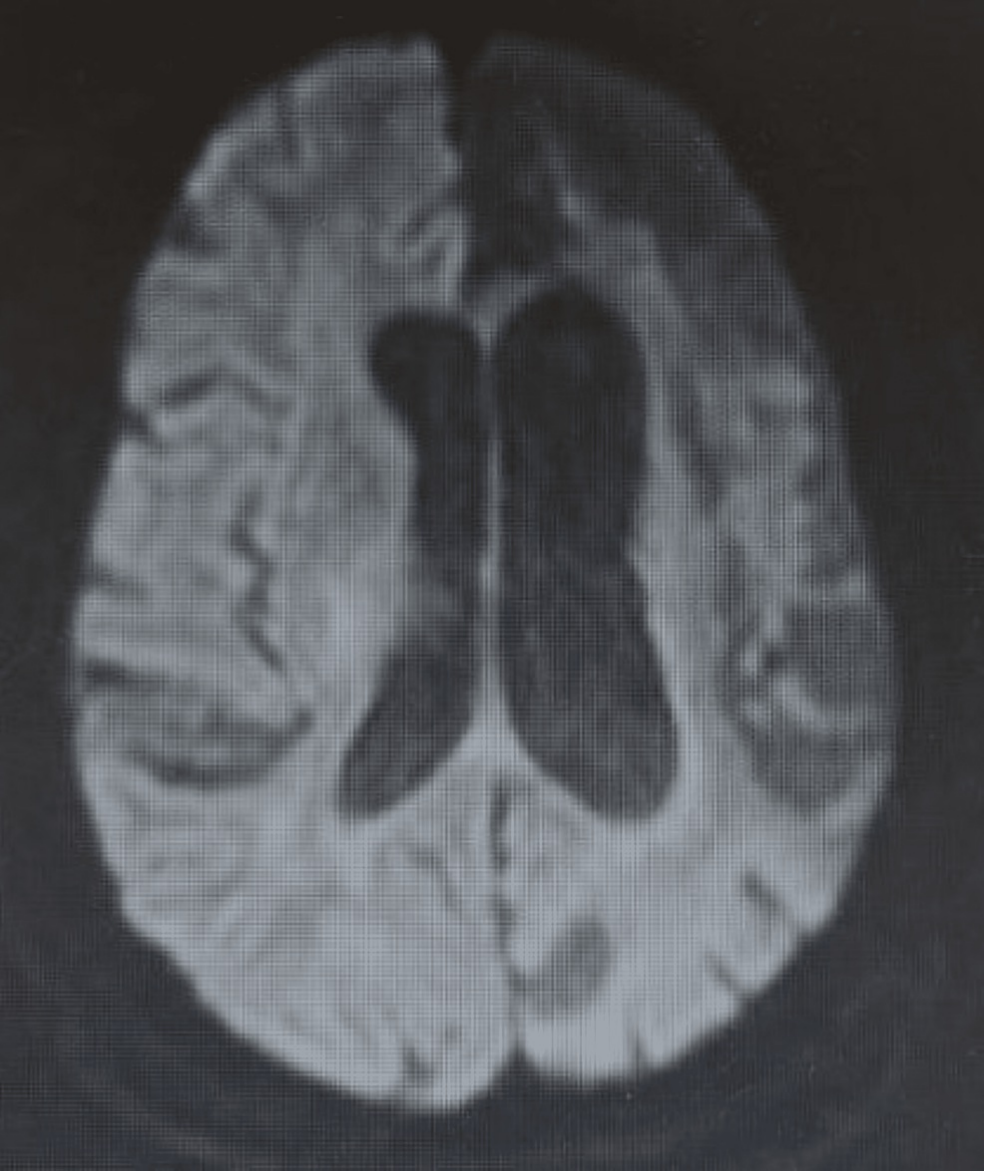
MRI DWI of the head shows no acute intracranial process. Large-volume encephalomalacia in the left cerebral hemisphere involving the ACA, MCA, and PCA territories. MRI DWI: magnetic resonance imaging with diffusion-weighted imaging; ACA: anterior cerebral artery; MCA: middle cerebral artery: PCA: posterior cerebral artery
